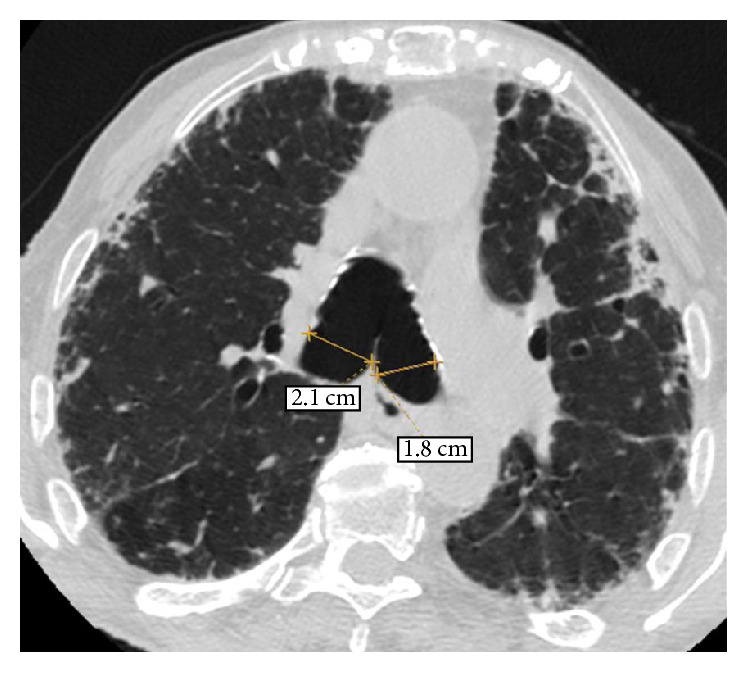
HRCT of the chest at the level of the carina. An enlargement of the right and left main bronchi is observed measuring in axial dimensions 2.1 cm and 1.8 cm, respectively. A subpleural reticular pattern associated with focal thickening of the pleura and traction bronchiectasis and bronchiolectasis is also noted.
